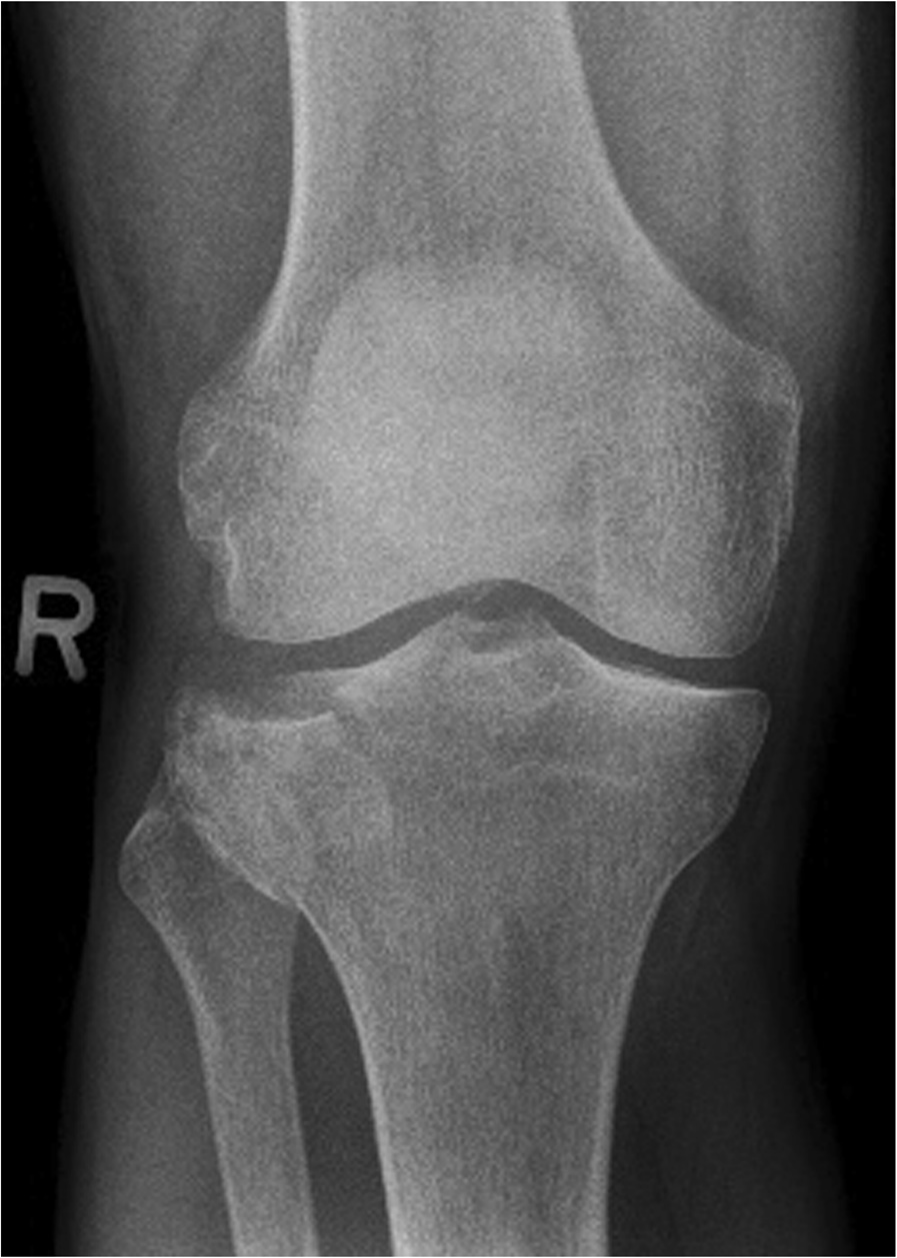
Anteroposterior (a-p) X-ray of the knee showing an AO 41 B3.1 fracture type.
